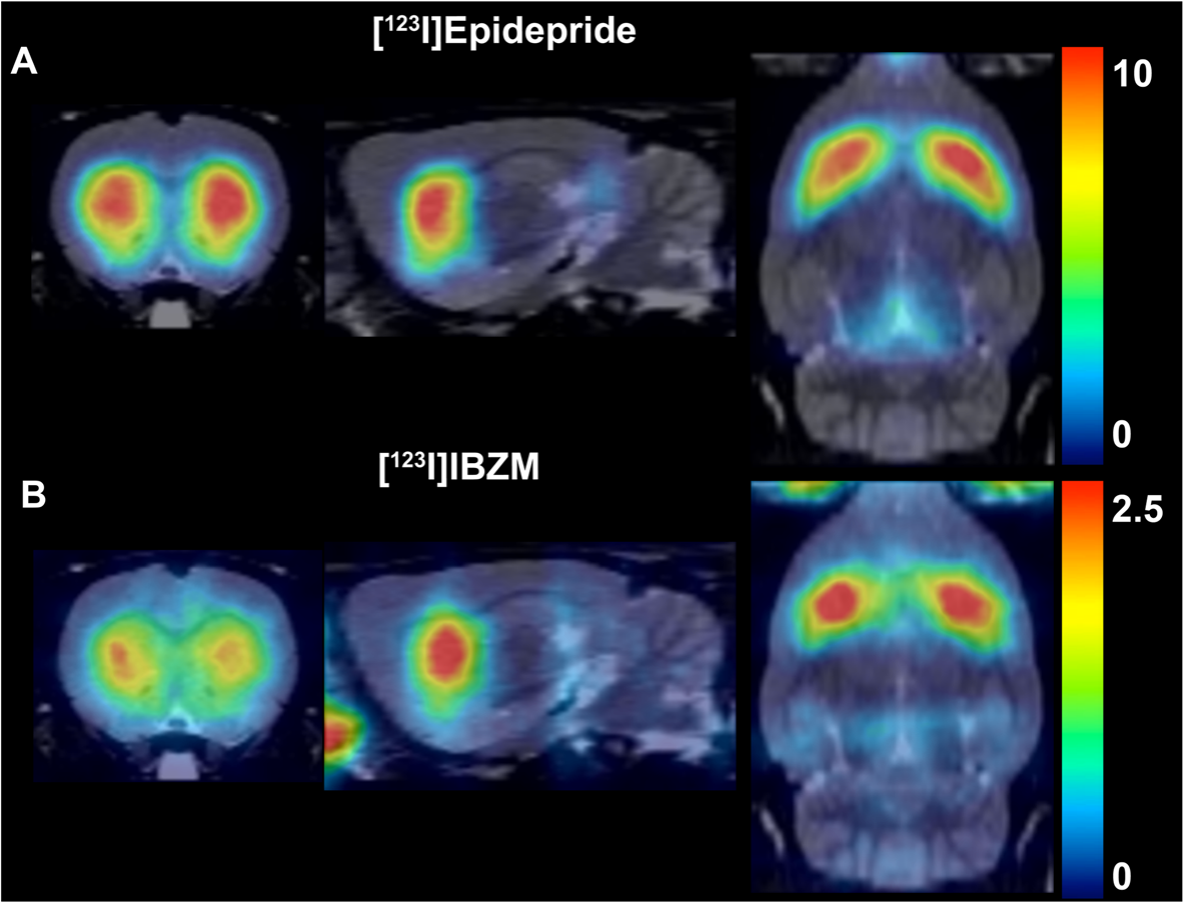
A SPECT image (coronal, sagittal, and axial planes) obtained with a [^123^I]epidepride and b [^123^I]IBZM. The color scale is in KBq/ml. Note the superior quality of the image obtained from the [^123^I]epidepride experiment in terms of a better anatomical delineation of the striatum and the highest binding of the radiotracer (the color scale of the [^123^I]epidepride image ranges from 0–10 KBq/ml, while the [^123^I]IBZM image ranges have a much lower binding, ranging from 0–2.5 KBq/ml)
